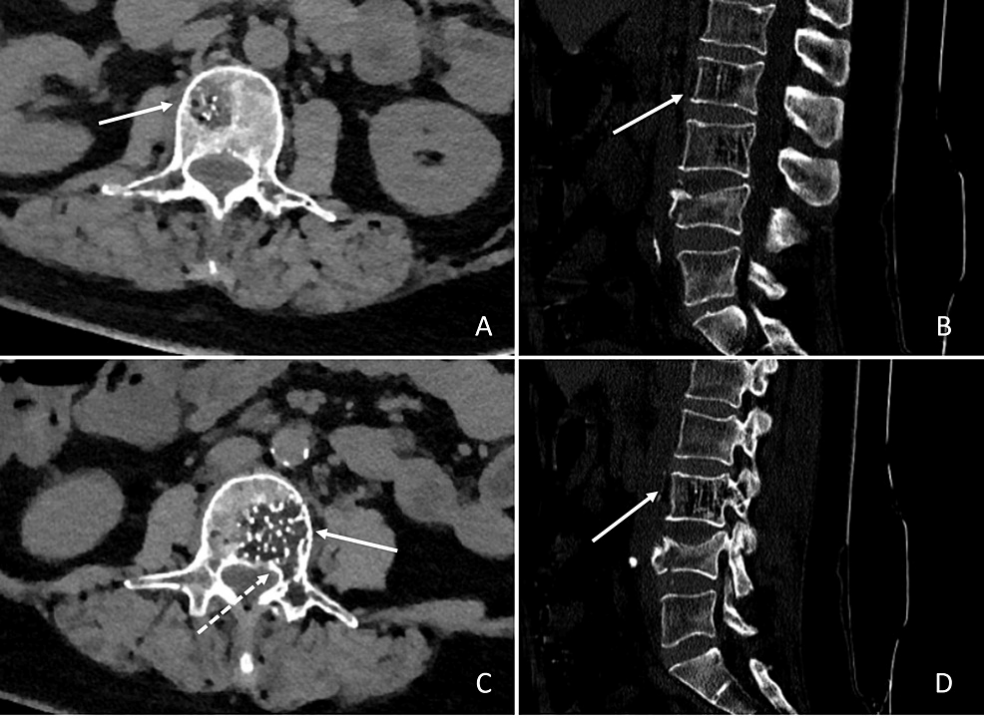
CT images of the patient There was evidence of lumbar adjacent atypical vertebral haemangiomas in L2 and L3 bodies. A. Axial image of L2. Microfractures were evident, as well as trabeculate structure (white arrow). B. Sagittal image of the lumbar column, paramedian right. C. Axial image of L3. The trabeculate pattern was evident on this scan (white arrow), as well as the involvement of the left peduncle (dotted white arrow). D. Sagittal image of the lumbar column, paramedian left. The “Honeycomb” structure was evident in the correspondence of the soma of L3 (white arrow).
